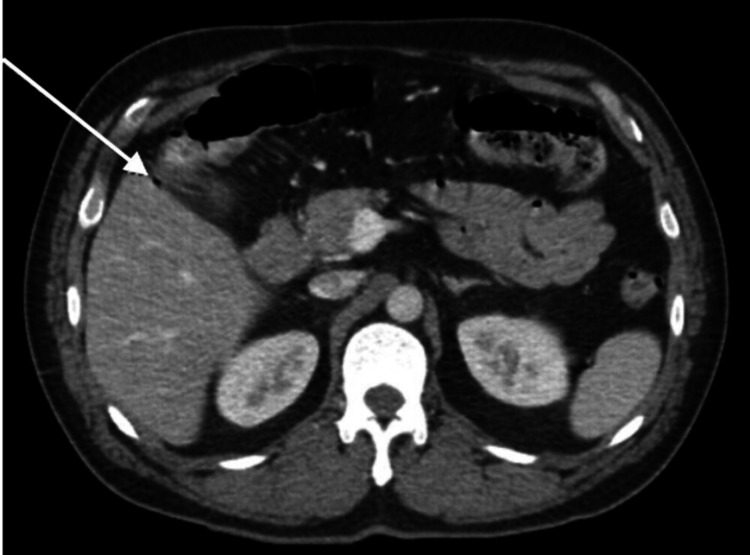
Axial view of the computed tomography scan of the abdomen, showing an intra-peritoneal air focus adjacent to bowel thickening, indicative of a micro-perforation at the site of endoscopic tattooing (arrow).
